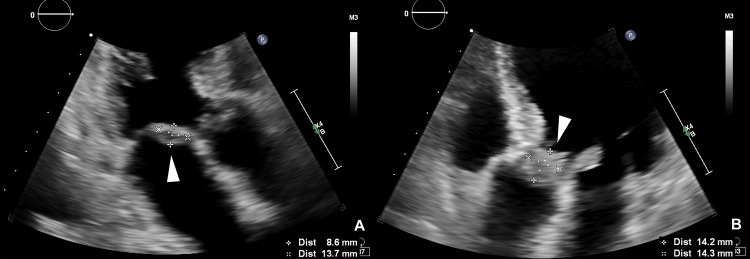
Initial TTE findings at admission (A-B) Vegetations are identified on both the mitral and aortic valves (white arrowheads). TTE, Transthoracic echocardiography
